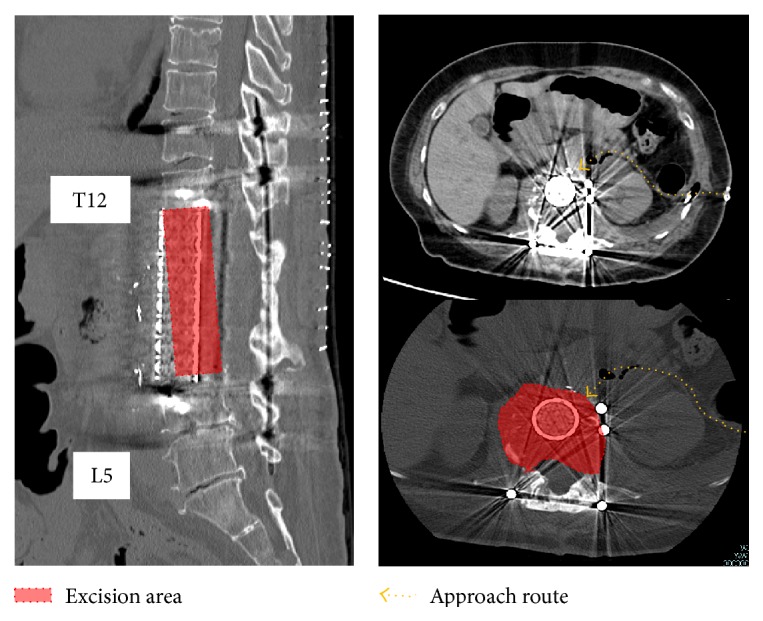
Postoperative CT images. The dashed line shows the approach route. The painted area indicates the excision area.
